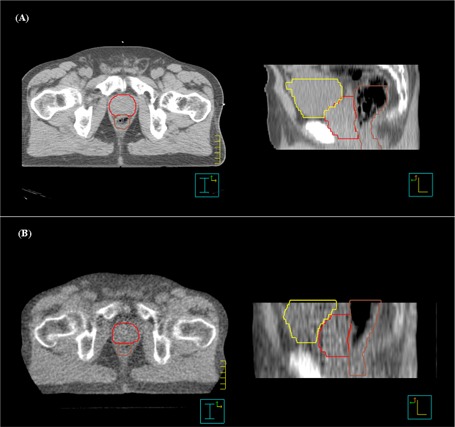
Comparison of (A) diagnostic computed tomography (CT) and (B) megavoltage CT images taken on the HI‐ART^2^ system (TomoTherapy, Madison, WI) for axial (left panel) and sagittal (right panel) views. The prostate, rectum, and bladder contours are shown. Note the superior–inferior displacement of the prostate relative to the reference treatment‐planning CT.
